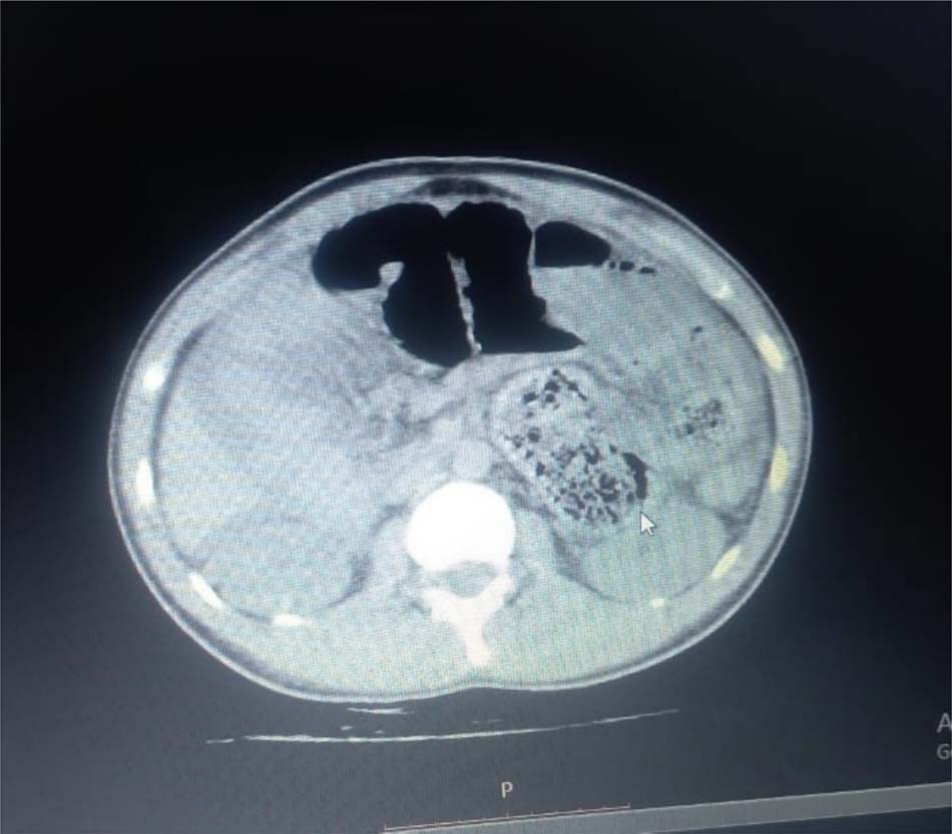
Axial CT demonstrating key pathological features. This contrast-enhanced axial view shows the colon displaced to the left side of the abdomen, confirming the presence of gut malrotation. The centrally clustered small bowel loops are seen encased in a dense sac, forming the characteristic cocoon structure that is pathognomonic for abdominal cocoon syndrome.
